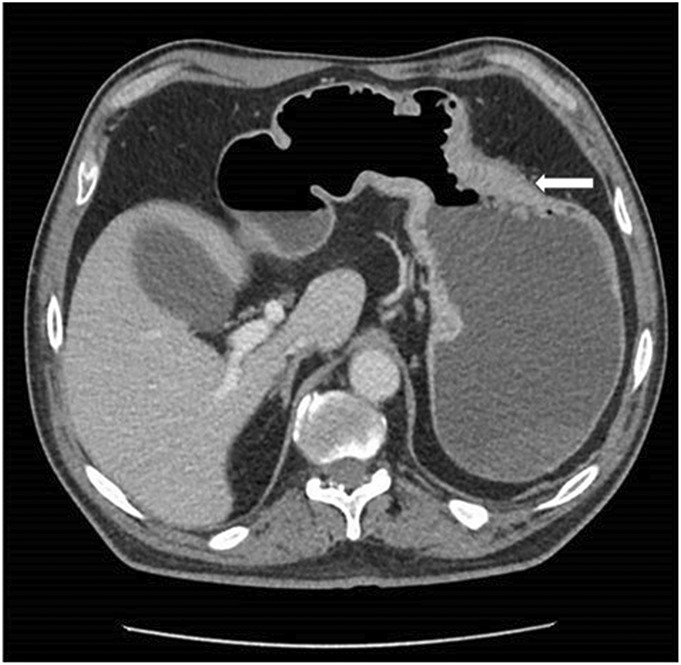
Computed tomography of the abdomen with dilated stomach and duodenum with decompressed distal small intestine (arrow).
